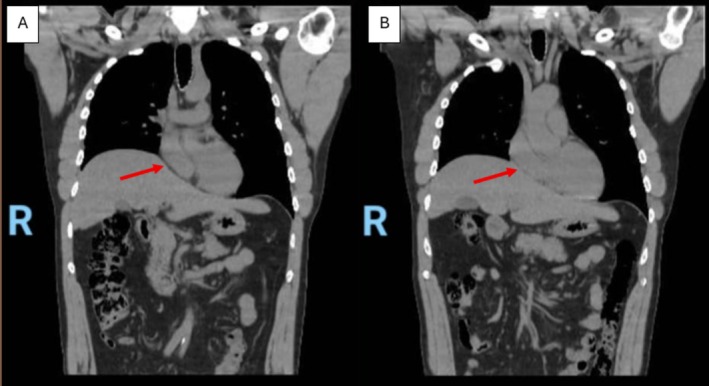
(Placeholder image for Video 2): Coronal view of the thoracic CT scan, demarcating a right hemidiaphragmatic eventration with the dome of the liver constricting the right atrium (arrow).
